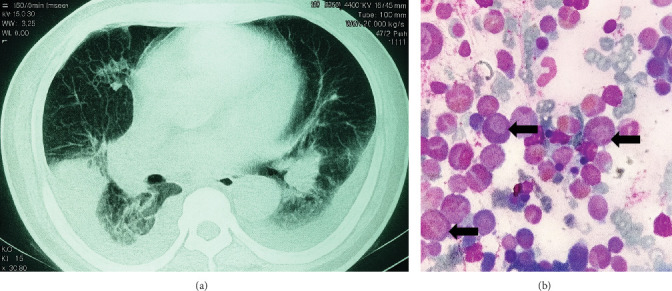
(a) Thoracic computed tomography showing the lungs with diffuse interstitial infiltrates and pleural effusion. (b) Bone marrow aspirate demonstrating myeloid hyperplasia (black arrows) and hypereosinophilia.
